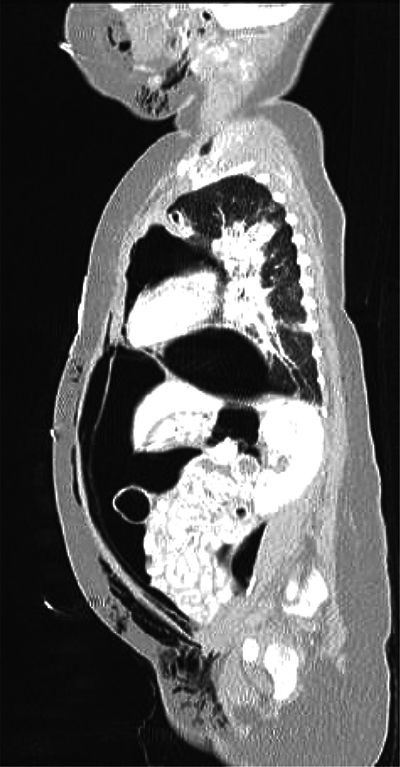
Whole body low-dose CT scanner. Latero-thoracic and right-cranial subcutaneous emphysema along with massive free air.
